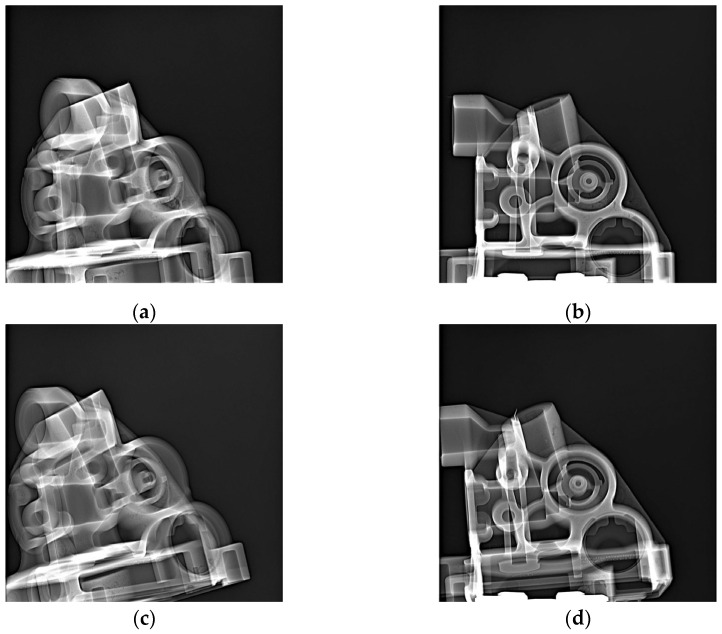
X-ray inspection for minimum (about 10 mm) value of biscuit thickness in specific sockets: socket C1: (a) area A—rate value 3, (b) area B—rate value 3; socket C2: (c) area A—rate value 3, (d) area B—rate value 3.
